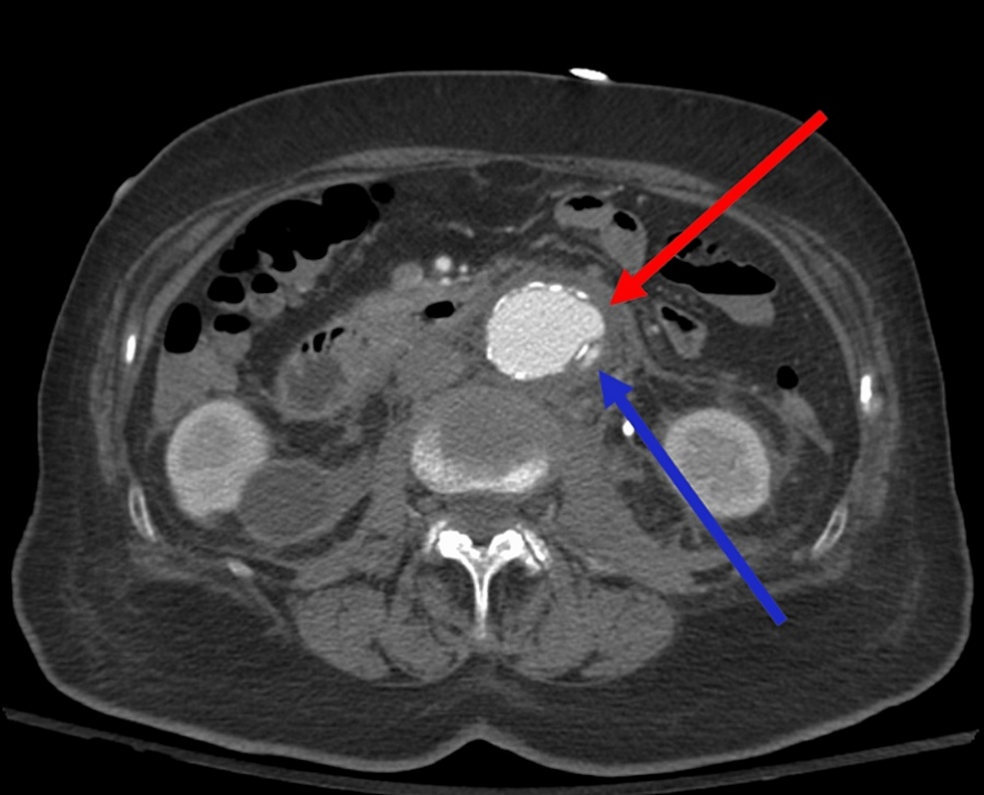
Computed tomography angiography (CTA) axial view, demonstrating aortic ulceration with pseudoaneurysm and intramural hematoma extending through the aortic wall
